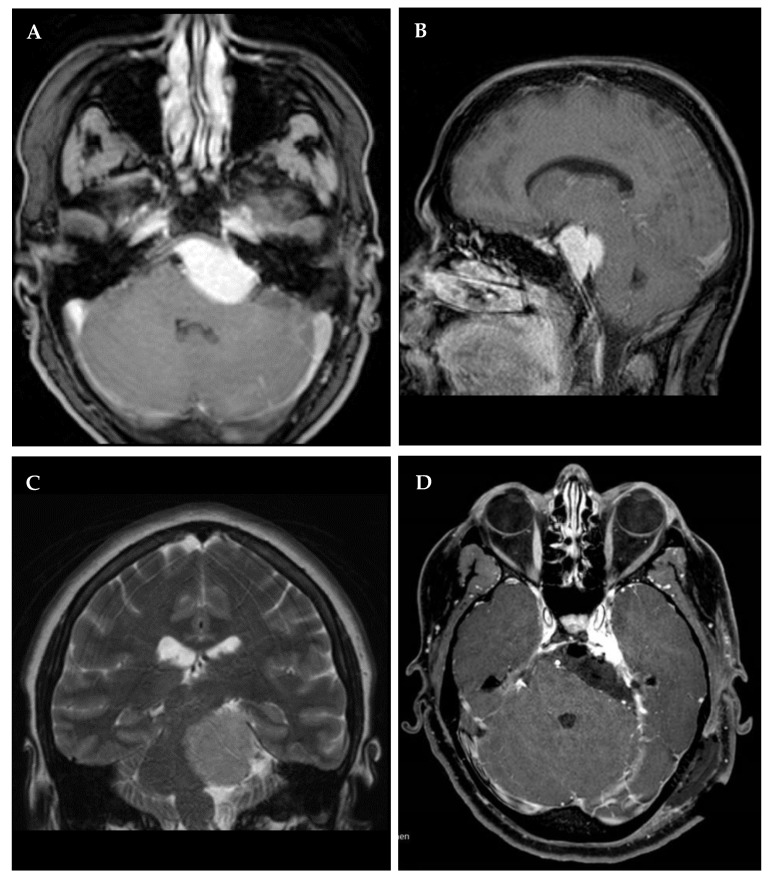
Left petroclival meningioma in a 31-year-old female who presented with trigeminal hypesthesia. Preoperative contrast-enhanced T1 magnetic resonance imaging (MRI) in axial (A) and sagittal (B) reconstructions as well as coronal T2 (C). Postoperative T1 MRI in axial reconstructions (D) after Simpson grade IV resection for decompression of the brain stem via a retrosigmoid approach.
